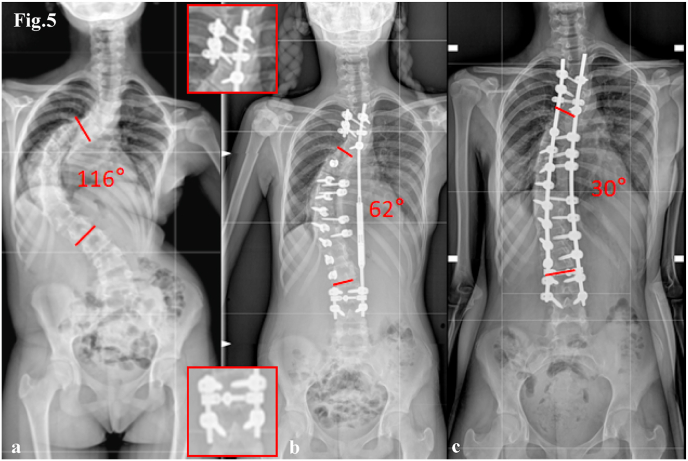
Case 2. 13-year-old female with a Lenke Type 1 curve, treated with T3–L4 posterior spinal fusion. Antero-posterior radiographs show: (a) preoperative curve, (b) correction with MCGR lengthening and proximal/distal “Sistema Quadro” construct, (c) final correction with definitive titanium/cobalt–chrome rods.
